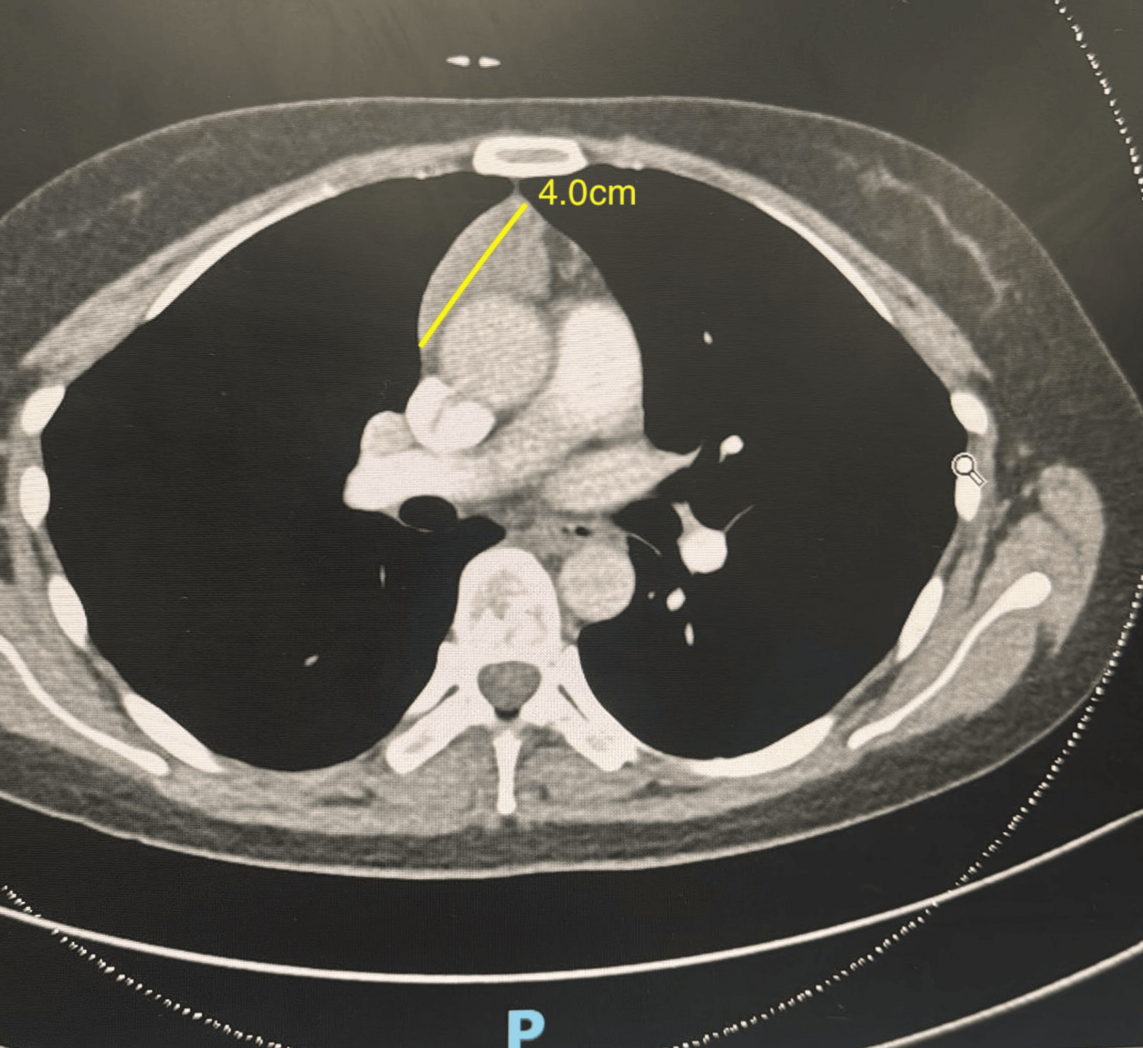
Initial CT image of the anterior mediastinal mass showing a triangular, homogeneous, hyperdense lesion without enhancing necrotic areas or lobulation.
